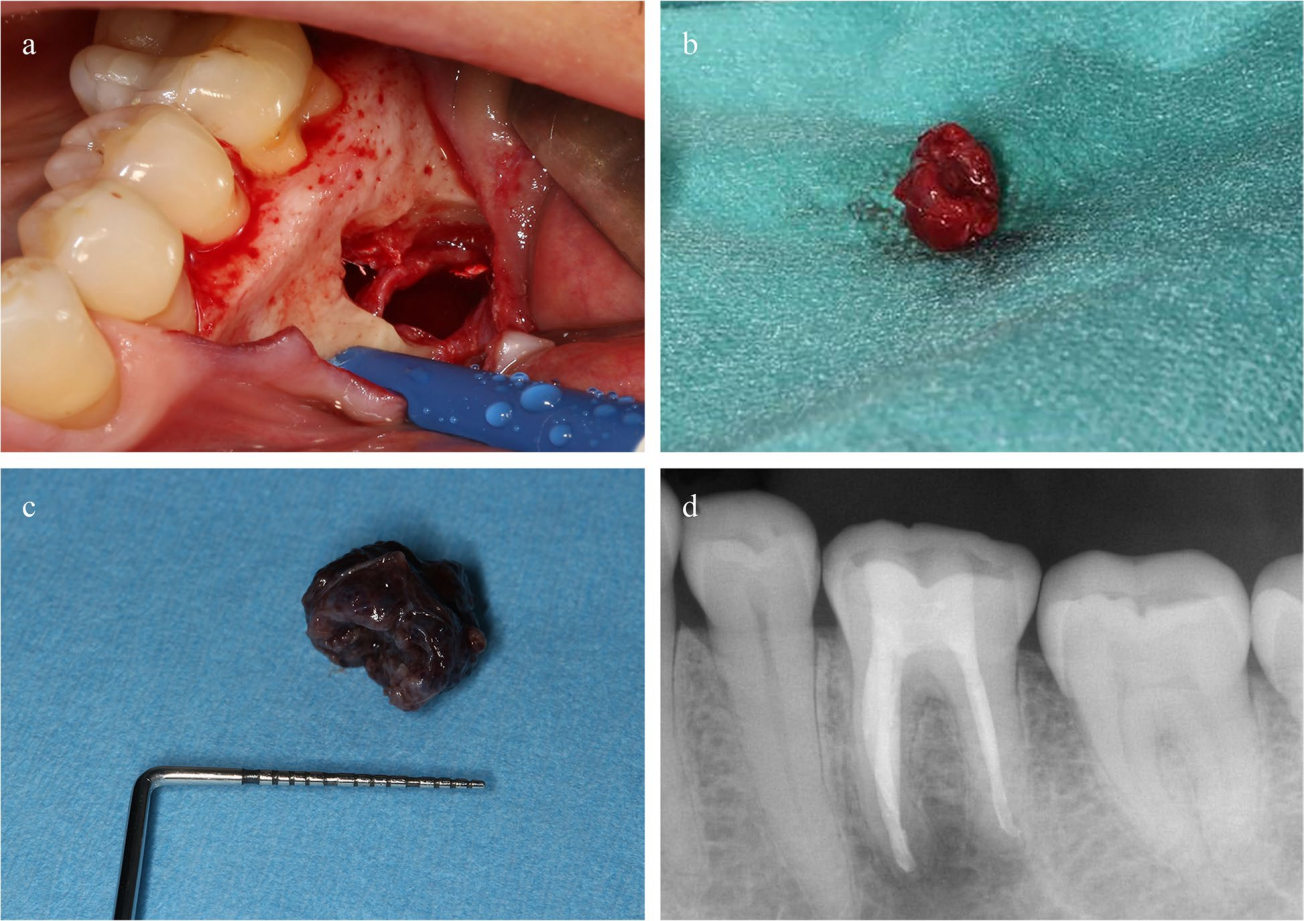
Intra/ extra-oral color photograph and periapical radiographs (Canon EOS 70D and Canon Macro Ring Lite MR-14EX, Canon, Tokyo, Japan). a Removed hemangioma. b Hemangioma after being fixed in formalin with the size of nearly 12 mm. c Clean intrabony cave depicting centrally the inferior alveolar nerve. d IOPAR after apicoectomy
